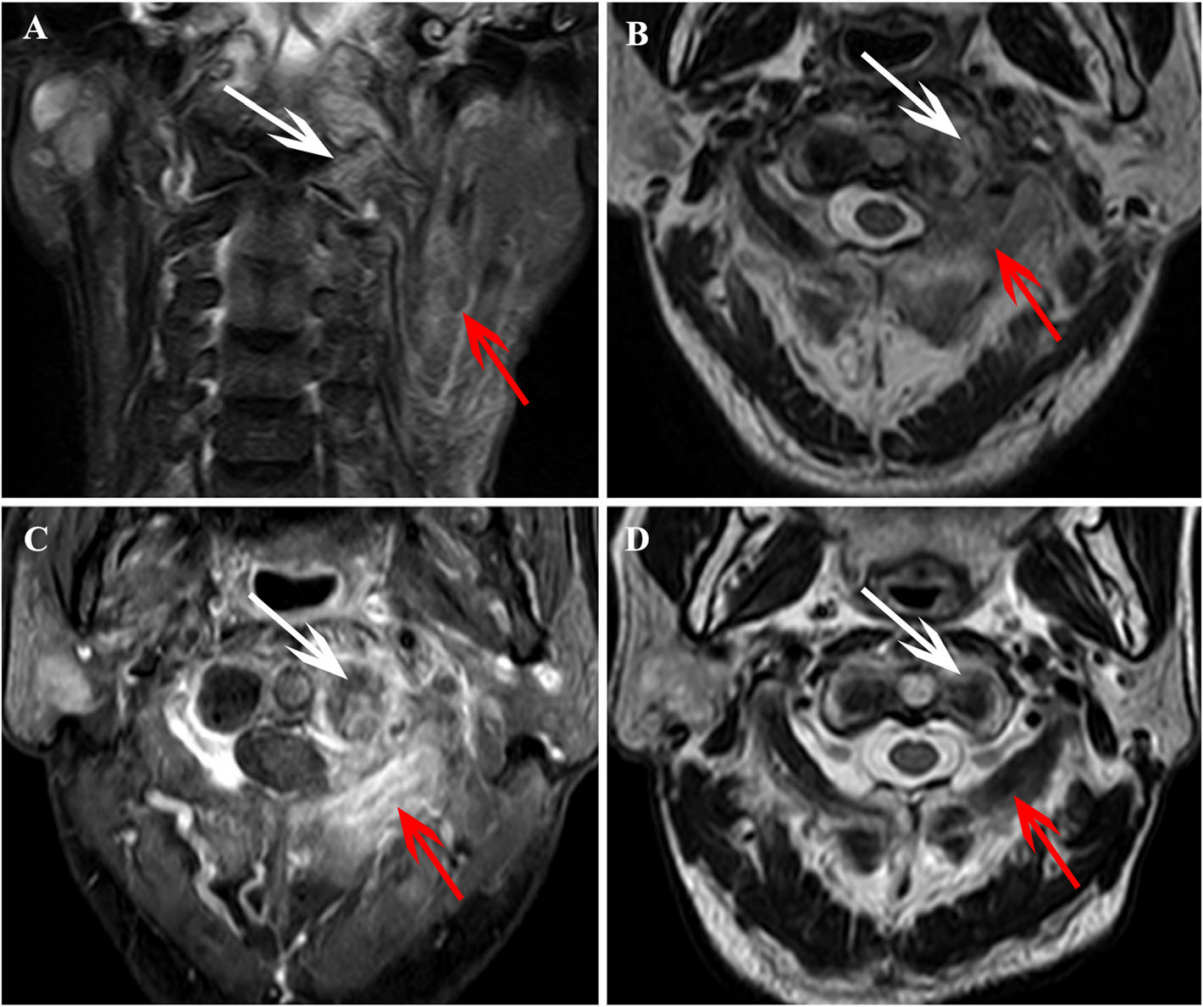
Images in a patient who was diagnosed with ORN of the cervical spine after radiotherapy for NPC, these images derived from our hospital, and patients’ informed consent was waived by the institutional review board. a Coronal FS T2-weighted image shows hyperintensity in the left aspect of C1 (white arrow) and shows muscle edema in the left neck (red arrow). b Axial T2W image shows hyperintensity in the left aspect of C1 (white arrow) and paravertebral muscle edema (red arrow). c Axial enhanced T1W image shows irregular endplate destruction and enhancement in the left aspect of C1 (white arrow), and shows marked enhancement in bilateral paravertebral muscles (red arrow). d At MRI follow-up examination after 10 months, the Axial T2W image shows the area of hyperintensity in the left aspect of C1 (white arrow) and paravertebral muscle edema (red arrow) has significantly shrunken
